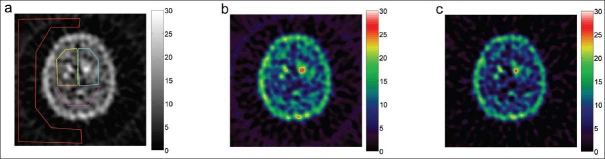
A slice of a reconstructed SPECT image for subject V (patient) in Table 1. The images shown correspond to those in Figure 3.
